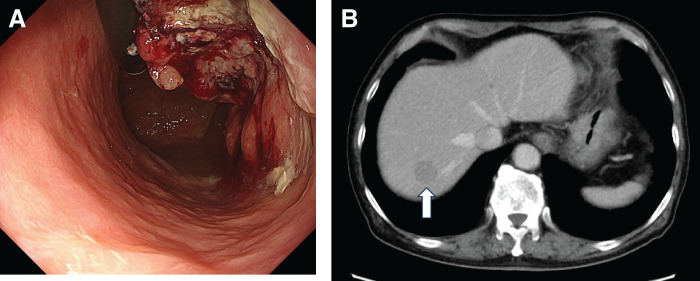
Pretreatment imaging findings. (A) Upper gastrointestinal endoscopy revealed a Borrmann type 2 gastric cancer on the anterior wall of the upper to middle third of the stomach. (B) Contrast-enhanced CT demonstrated a 2.2-cm liver metastasis in segment 8 (S8) (white arrow).
